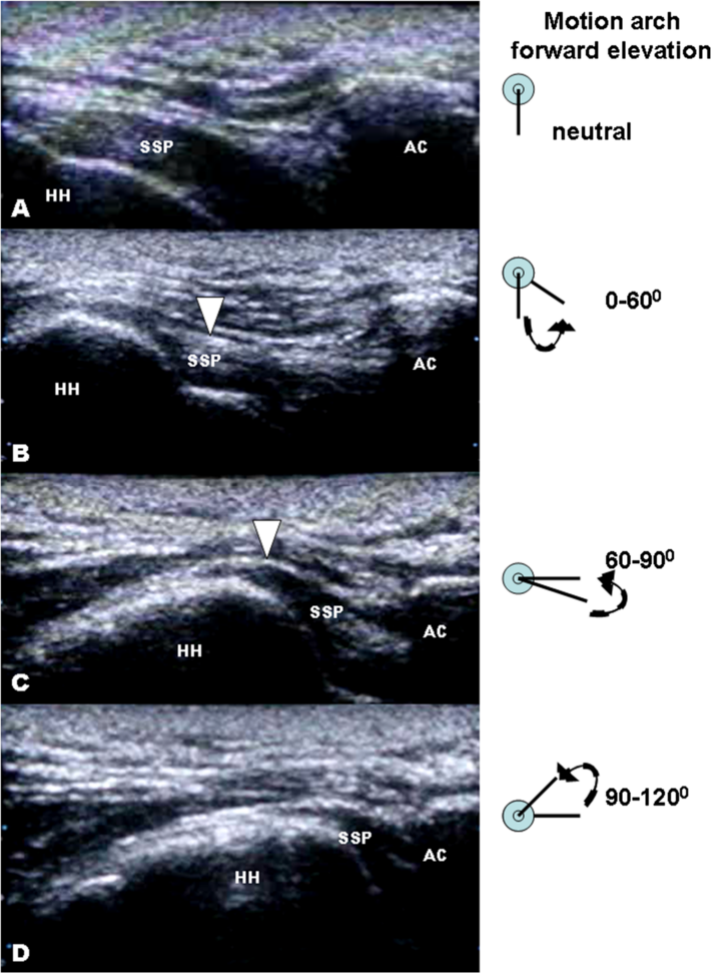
Sonographic findings with shoulder impingement. Dynamic musculoskeletal sonography of a 36-year-old man developed shoulder impingement syndrome after receiving hook plate fixation of a left distal clavicle fracture. The treated shoulder was passively and forwardly elevated from a neutral position towards 180° elevation. It was stopped at 120° when the patient reported intolerable pain. (A–C) At 90° forward elevations, bunching of supraspinatus tendon fibers (arrowheads) was noted accompanied with distention of the subacromial/subdeltoid bursa (arrow) signifying flowing fluid of bursitis. (D) Another remarkable finding is abnormal superior translation of humeral head with regard to the acromion obstructing its passage beneath the acromion. SSP, supraspinatus tendon; HH, humeral head; AC, acromioclavicular joint.
